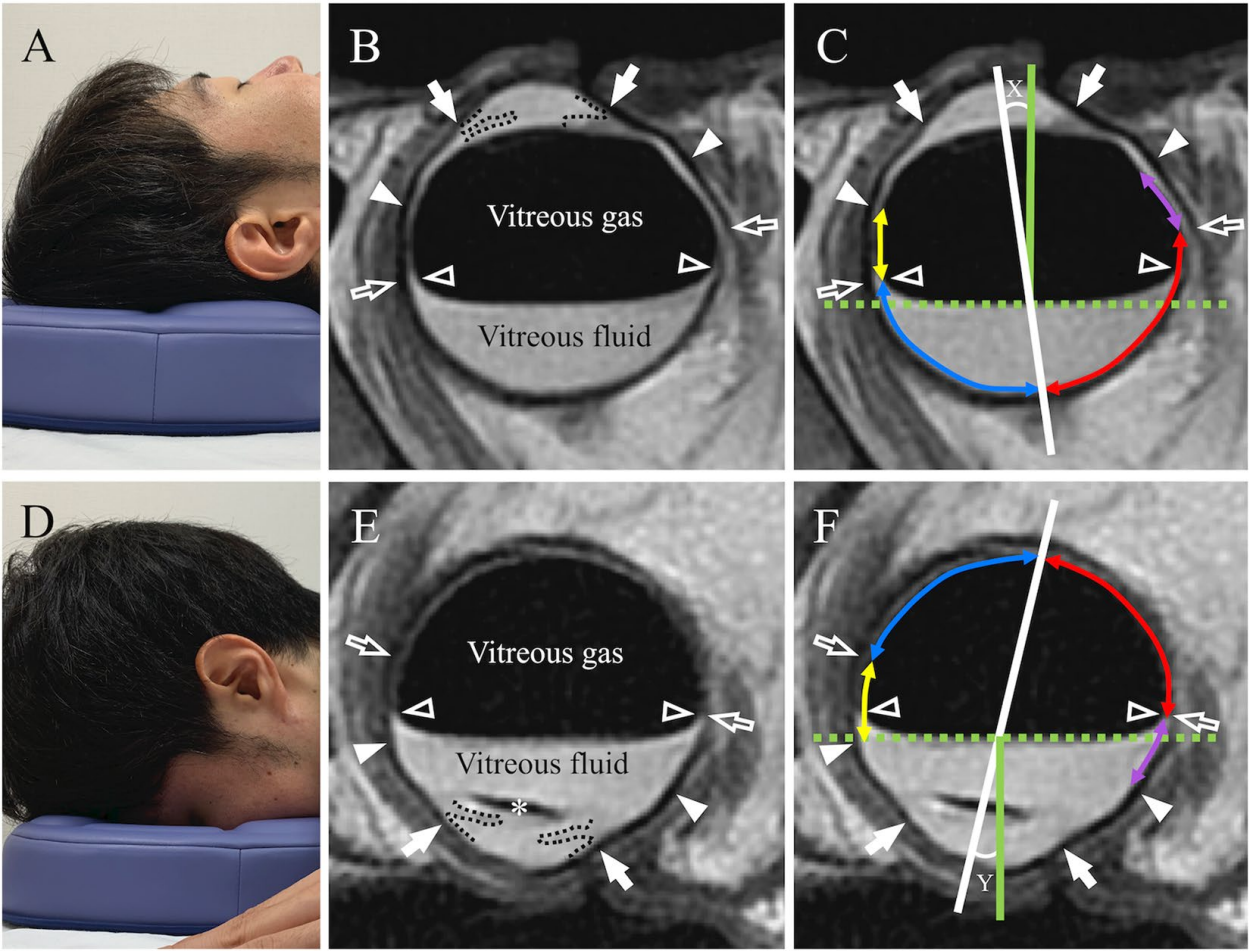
Positions during MRI examination and MRI images in the supine and prone positions. The supine (A) and prone (D) positions during MRI; (B,C,E,F) MRI images of case 5; (B,C) in supine position and (E,F) in prone position. White arrows, white arrow heads, and white open arrow indicate corneal limbus, ora serrata (7.0 mm posterior to the limbus) and equator line (13.5 mm posterior to the limbus), respectively. White open arrow head indicates the ends of the retina contacting with the gas. Black dotteds line indicate the margin of angle and iris. IOL could be observed (*). White solid line, green dot line, and green solid line indicate pupillary axis, horizontal line, and perpendicular line to the ground, respectively. X and Y are the supraduction angle to the perpendicular line in supine and prone position, respectively. The anterior part to the equator is shown as purple and yellow line, which indicates the inferior–anterior and superior–anterior parts of the retina, respectively. The posterior part to the equator is shown as red and blue line. Red and blue lines indicate the inferior–posterior and superior–posterior parts of the retina, respectively.
